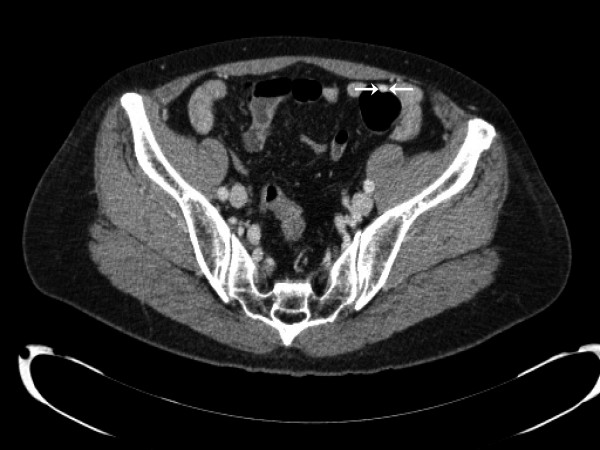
Radiologic characterization of the lesion. CT imaging revealed a highly enhanced intramural mass (1.3 cm in maximum diameter; white arrows) in the proximal sigmoid colon.
